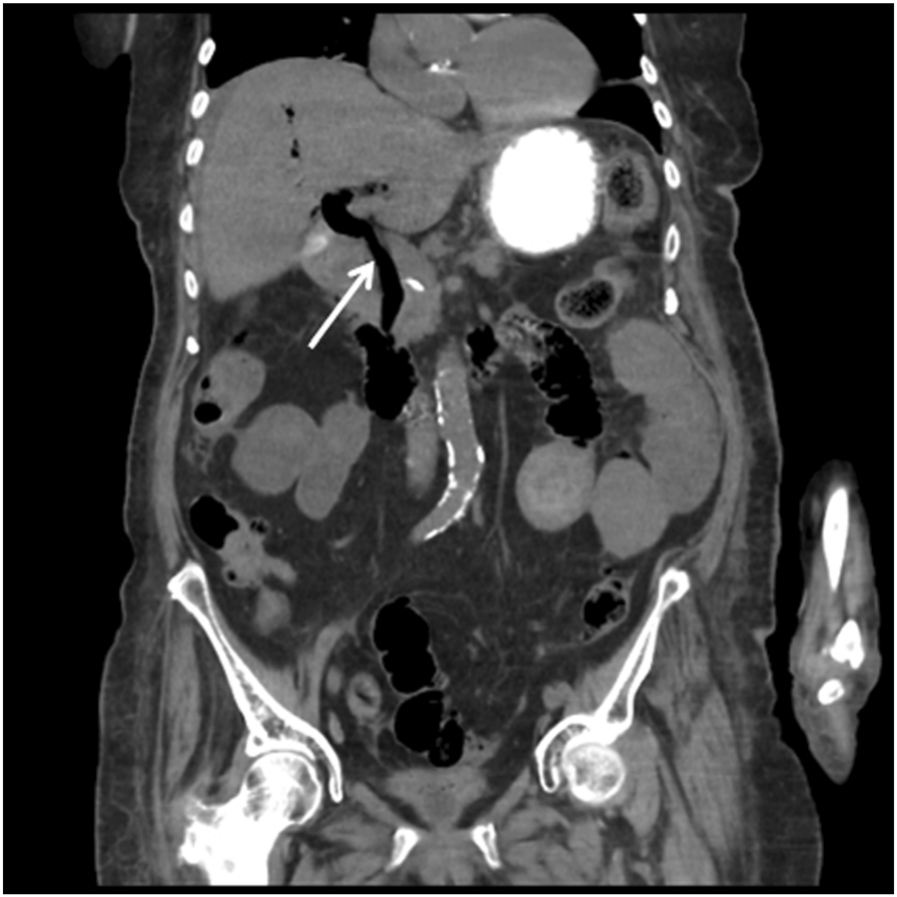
Computed tomography image (coronal view) showing a cholecystoduodenal fistula (arrow).
